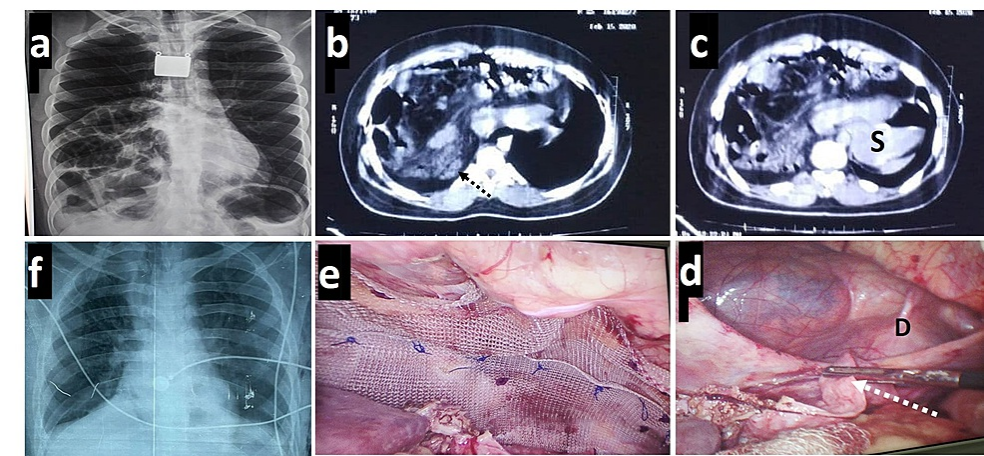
Clockwise from upper left: (a) Pre-operative chest radiograph with multiple viscera in the right hemithorax. (b) Cross-sectional contrast CT suggestive of multiple viscera in the right hemothorax and a linear hyperdensity (marked with dashed black arrow) possibly representing the atrophied left liver. (c) Similar CT image showing the stomach (marked S) entering the thorax through the defect and falling to the left. (d) Operative image with the large defect (D) with indistinct ventral edges. The dorsal edge is being cleared by dissecting away the atrophied left liver (marked with dashed white arrow). (e) Defect closure and a large overlapping mesh has been placed. (f) Postoperative chest radiograph with complete lung expansion on day seven. CT - computed tomography.
